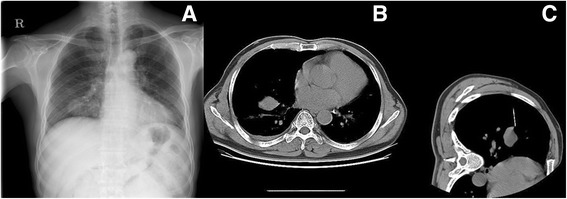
Chest radiograph on first diagnostic period. (a) Shows the mass shadow occupying middle and lower zone of right hemithorax. (b) 3.9 × 3.6 cm sized intrathoracic mass in right lower lobe lung field on Chest CT. (c) Biopsy CT during transthoracic needle biopsy
